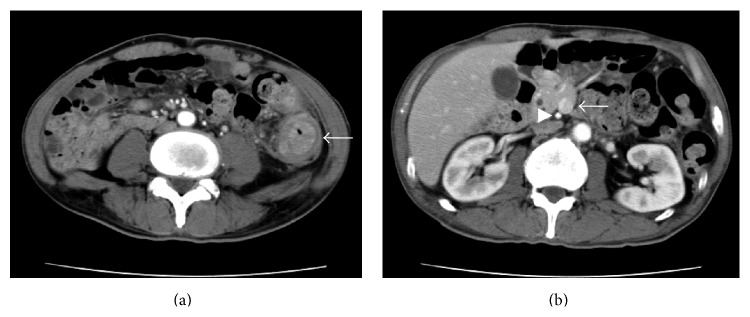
Computed tomography (CT) of the abdomen revealed thickening of the descending colon wall, which was indicative of advanced descending colon cancer (arrow) (a); superior mesenteric vein rotation was also observed (arrow) (b).
